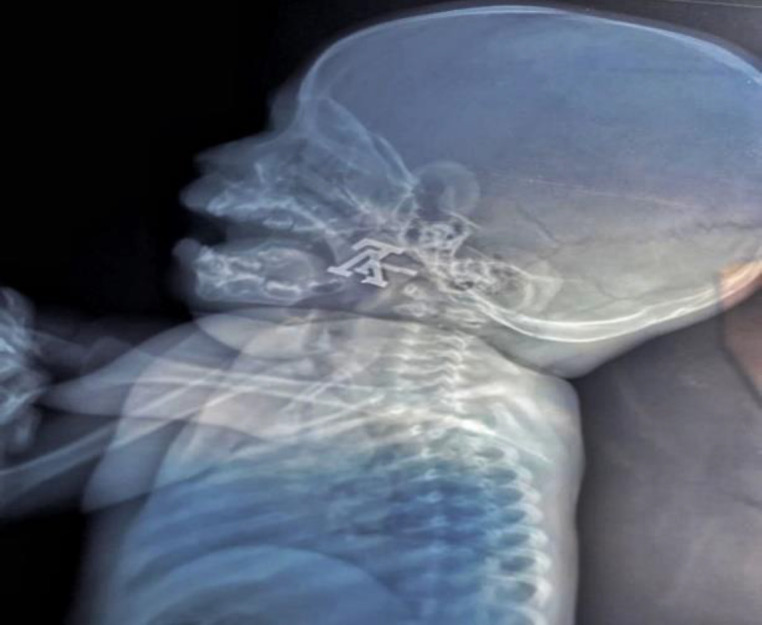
X-ray soft tissue neck (lateral view) showing radio-opaque foreign body in nasopharynx
